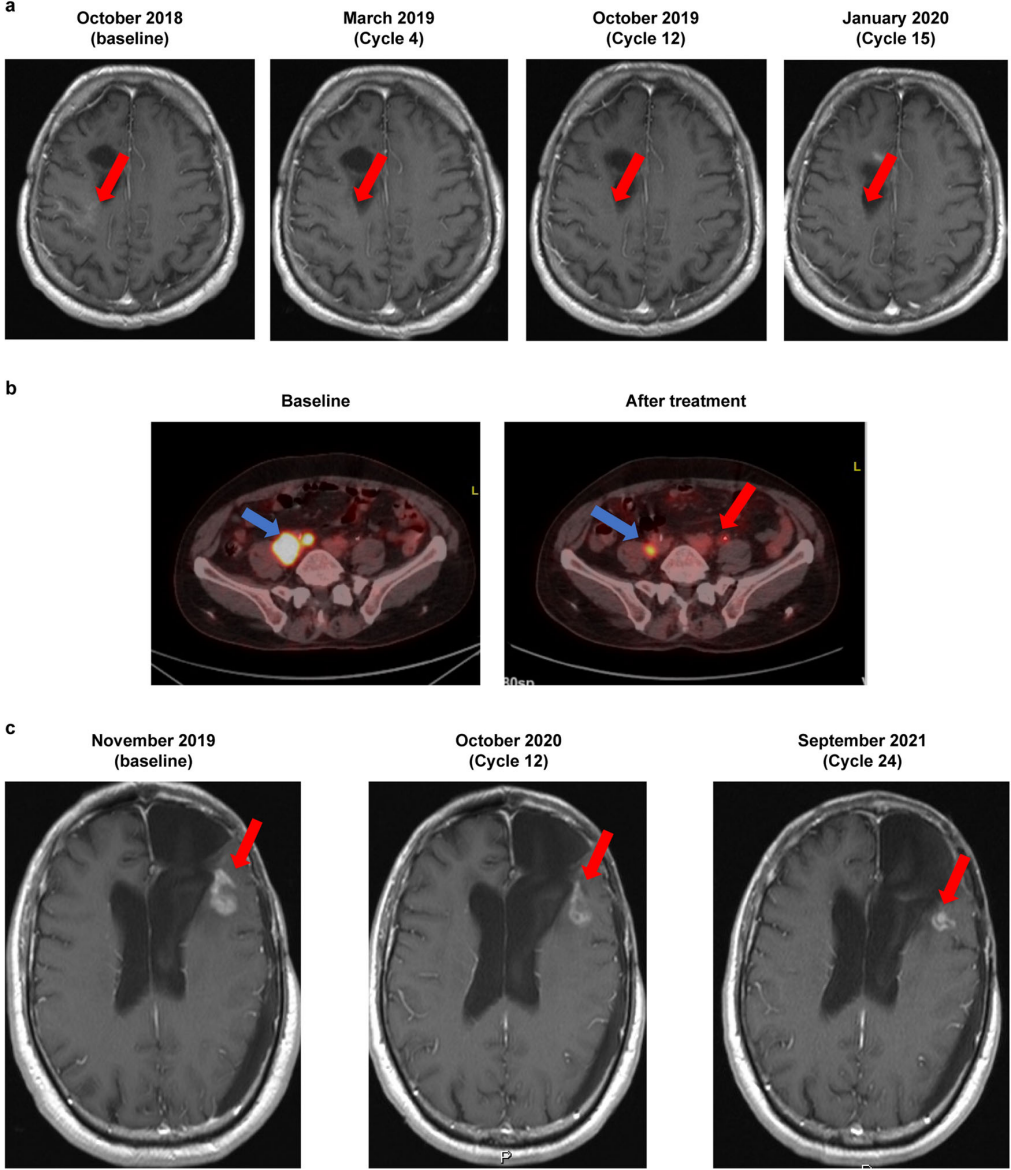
Imaging results of representative responses to trotabresib. a MRI evaluation of a patient with grade 2 astrocytoma. The patient had a complete response on trotabresib (red arrows), with the disappearance of both enhancing and non-enhancing areas compared with baseline. The patient discontinued after 19 cycles of treatment due to disease progression. b FDG-PET/CT evaluation of a patient with germinal center B-cell immunophenotype R/R DLBCL who had a 57% reduction in target lesion size after two cycles of trotabresib (blue arrow). This was accompanied by the appearance of a new metabolic lesion (red arrow), and treatment was discontinued after four cycles of trotabresib due to disease progression. c MRI evaluation of a patient with refractory high-grade astrocytoma. The patient had a minor response on trotabresib with a 38% reduction in tumor size (red arrows), and the patient remained on study treatment at cycle 34 with a continued response as of June 16, 2022. *CT* computed tomography, *DLBCL* diffuse large B-cell lymphoma, *FDG* fluorodeoxyglucose, *MRI* magnetic resonance imaging, *PET* positron emission tomography, *R/R* relapsed/refractory. The two left-most images in panel a are reprinted from *Annals of Oncology* 31(6), Moreno V, et al. Phase I study of CC-90010, a reversible, oral BET inhibitor in patients with advanced solid tumors and relapsed/refractory non-Hodgkin’s lymphoma, Page 780–788, Copyright 2020, with permission from Elsevier.
